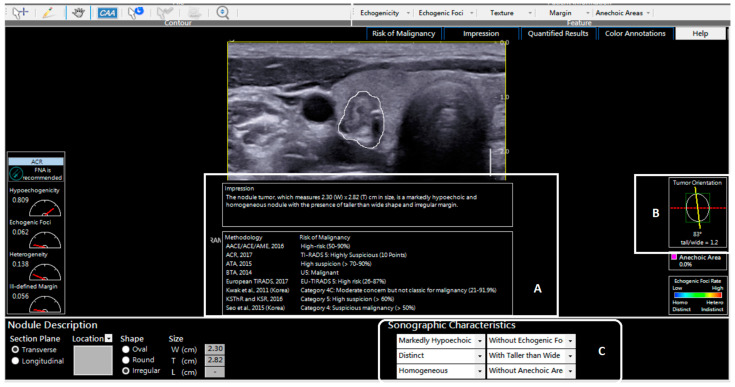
An example of a typical image analysis output from the thyroid CAD software. (A): The malignancy risk stratification of the nodule based on different classification systems. (B): The software’s computation of the taller-than-wide ratio. (C): The software’s computation of the sonographic features based on the analyzed image.
